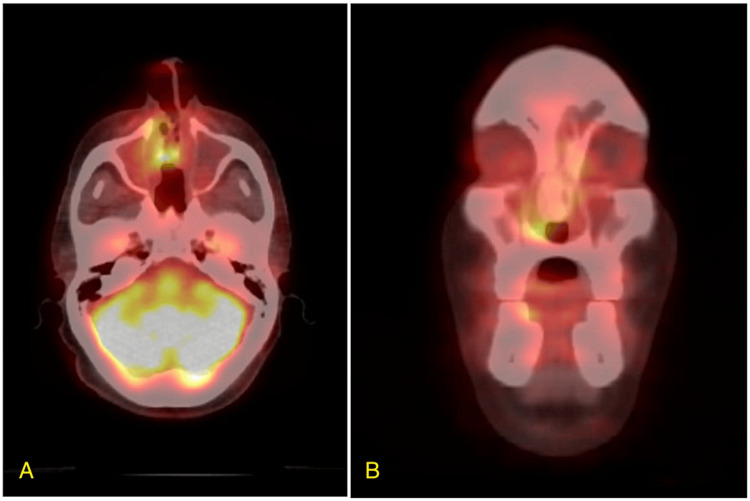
Three-month post-treatment axial (A) and coronal (B) PET/CT images
